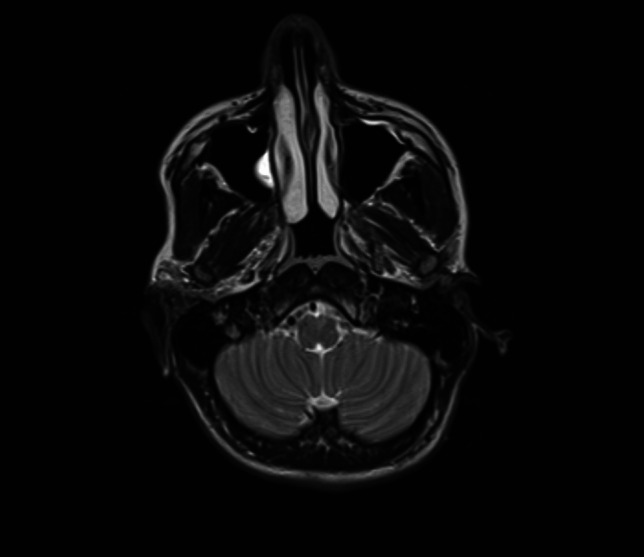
MRI of the head image. Small area (size 5 × 2.5 mm) of ​​discreetly increased T2WI/FLAIR signal, without diffusion restriction, without pathological contrast enhancement corresponding to gliosis after an ischemic episode
